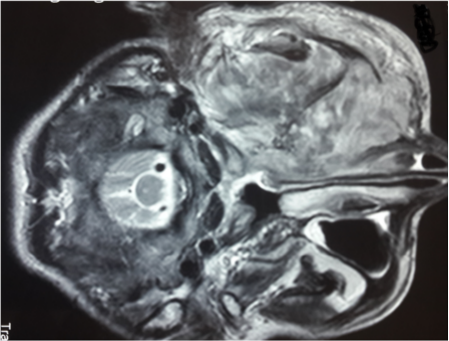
Axial T2-weighted magnetic resonance image of the facial area showing the ethmoidonasal, and intraorbital extension, with infiltration of the jugal and temporozygomatic soft parts
